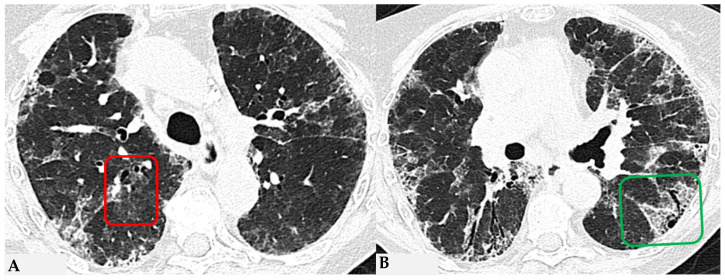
FHP with small areas of GGO related to acute exacerbation: axial chest HRCT scan. HRCT pattern characterized by mild amorphous GGO components in the upper lobes (red box), predominantly centrally located, with sectoral signs of interface and anterior asymmetric reticulations. Minimal traction bronchiolectasis coexists (A); subcarinal scan reveals GGO with moderate distortion, septal thickening and alterations predominantly bronchocentric in distribution (green box in (B)). The changes exhibit a “patchy” distribution with areas of mosaic attenuation.
